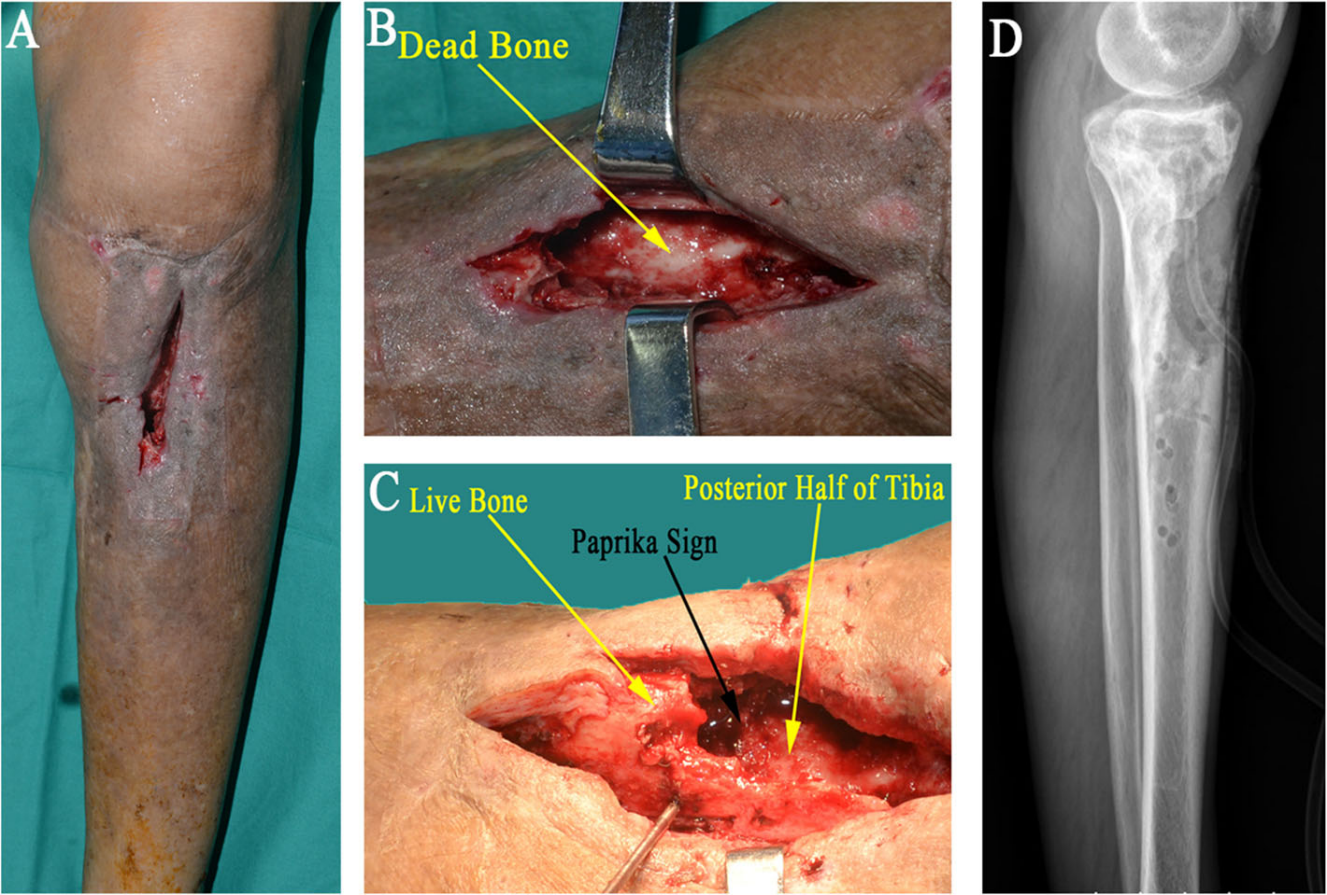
Debridement technique. a Clinical photograph of the affected leg. b, c During debridement, the infected or necrotic bone is removed radically until the “paprika sign” (cortical bone bleeding area) appears. It is of great importance to make sure that the posterior half of tibia retained following the radical debridement is uninfected and intact. d Lateral radiograph shows bone defect following resection of dead bone
